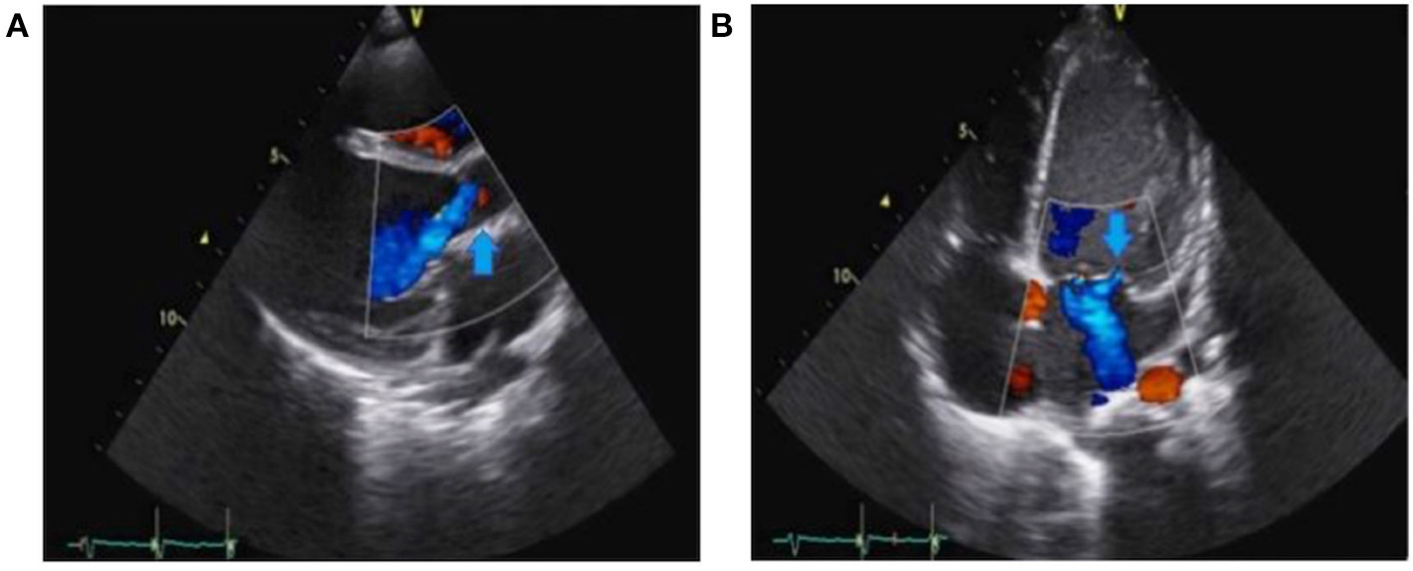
Preoperative transthoracic echocardiography (TTE) showing severe aortic regurgitation (arrow) in (A) and severe mitral regurgitation (arrow) in (B).
